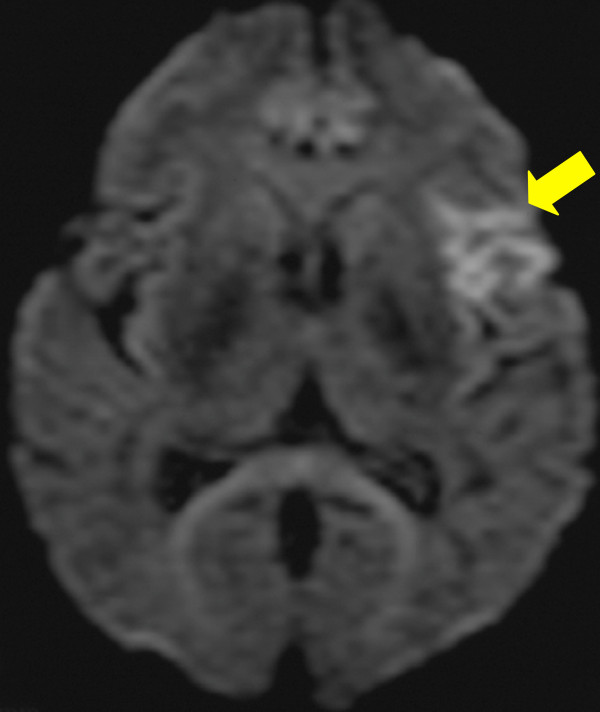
Diffusion-weighted MRI scan. Yellow arrow indicates the middle cerebral artery areas of high signal intensity.
